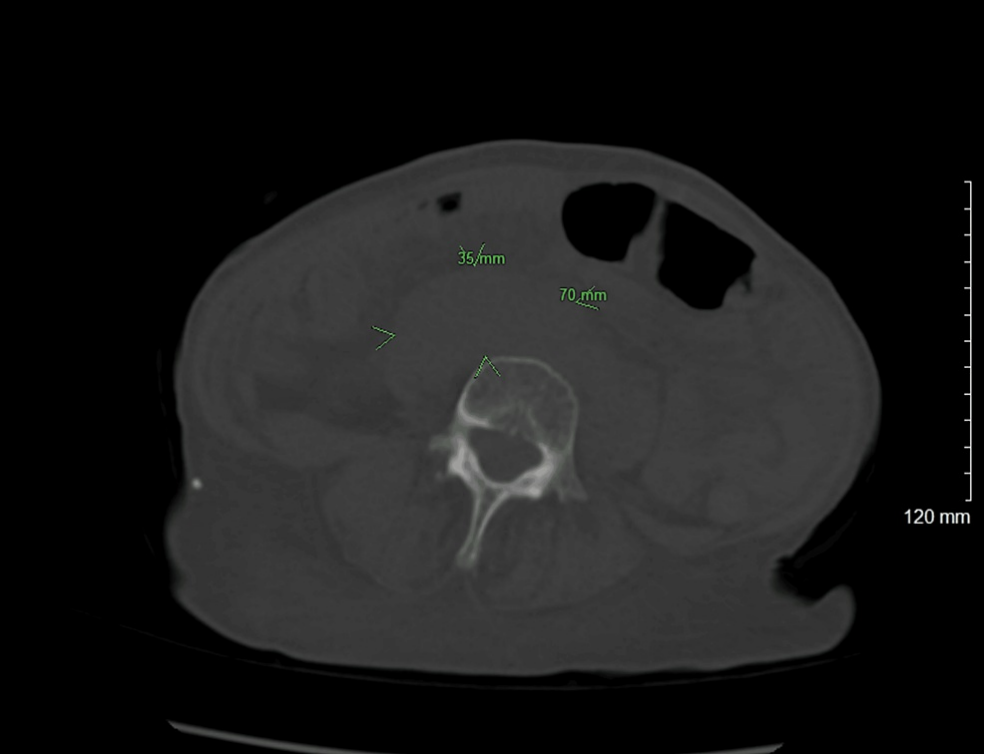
Non-contrast computed tomography of the pelvis demonstrating 7×3.5 cm right retroperitoneal mass (green arrowhead)
